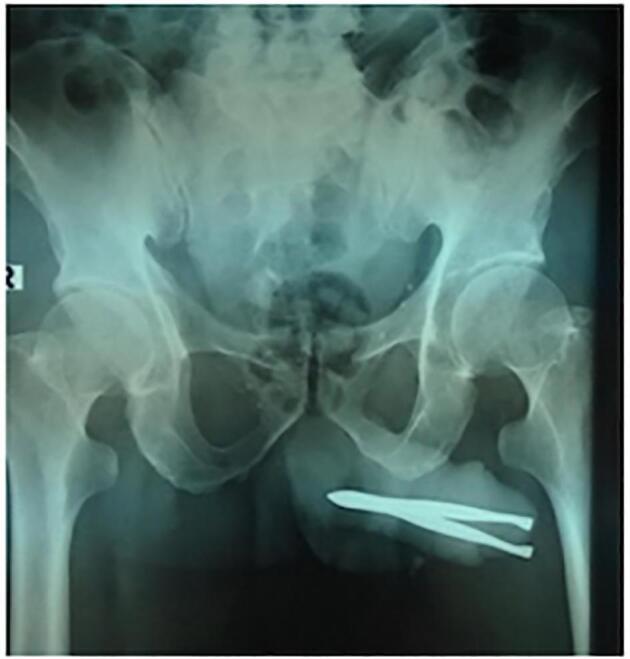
Pelvic X-ray, tweezers in the urethra.
